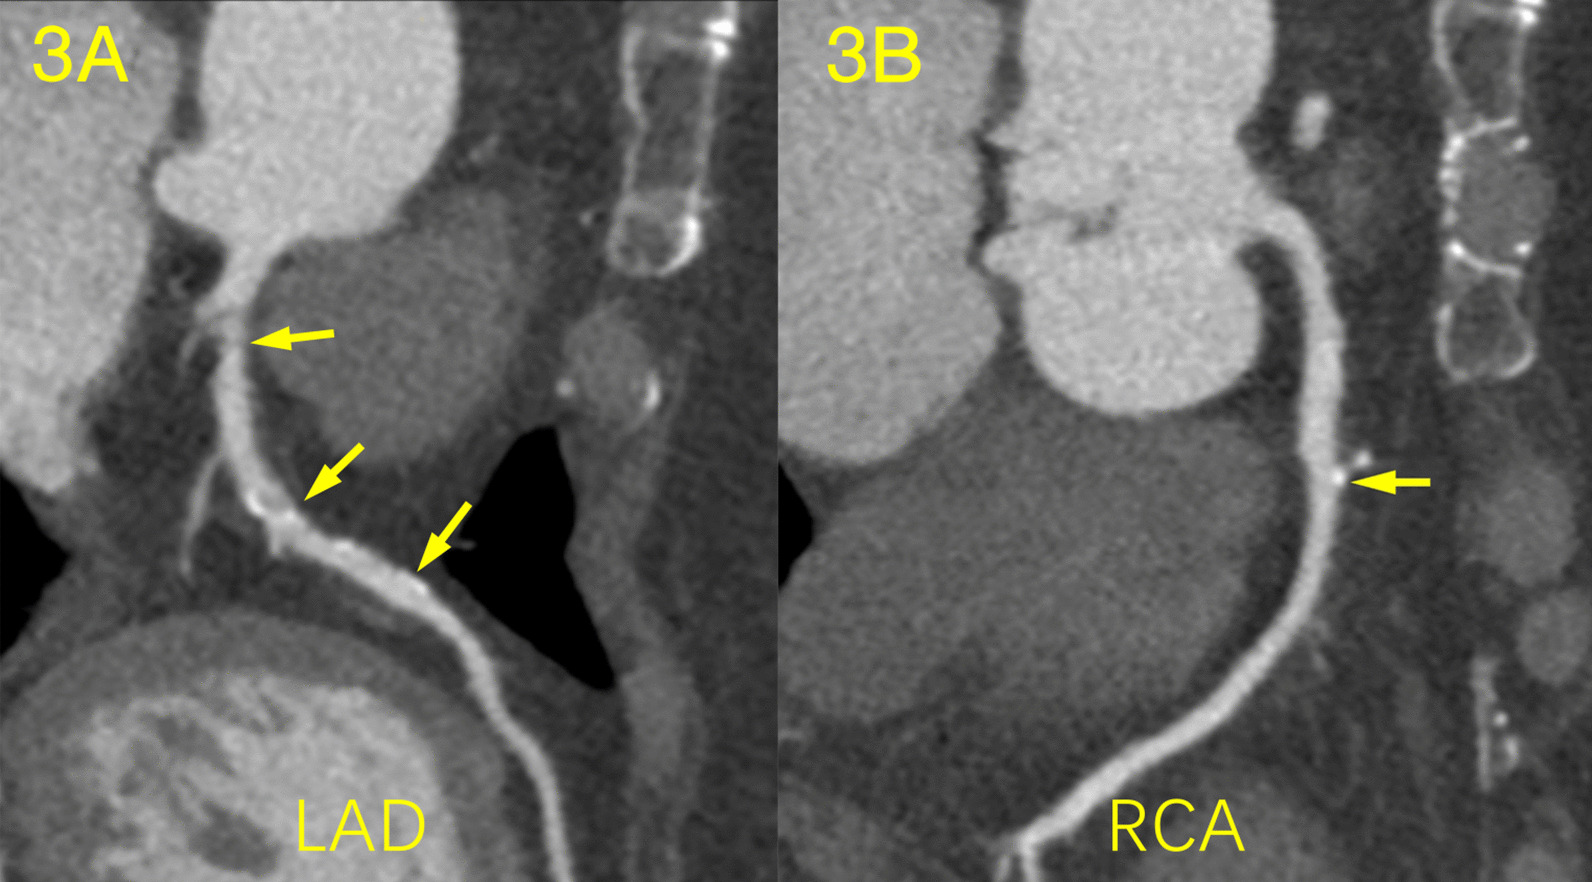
Calcified and mixed plaque in a 71-year-old woman with mild SCH and DM. The patient underwent coronary CTA for palpitations. a Curvature planar reconstruction CT image of the LAD artery shows several mixed plaques in the proximal and middle segments of the LAD with mild stenosis. b The image of the RCA artery shows small calcified plaque in the middle segment of the RCA with minimal stenosis
